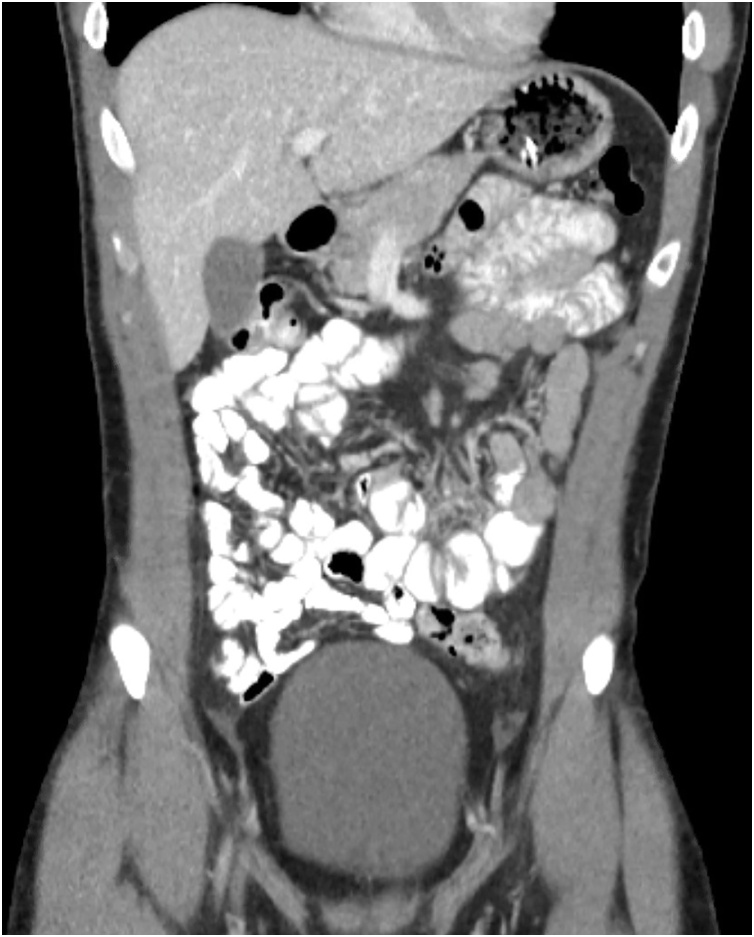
Coronal view of CT abdomen and pelvis with PO contrast after 24h of non-operative management of SBO*.*
